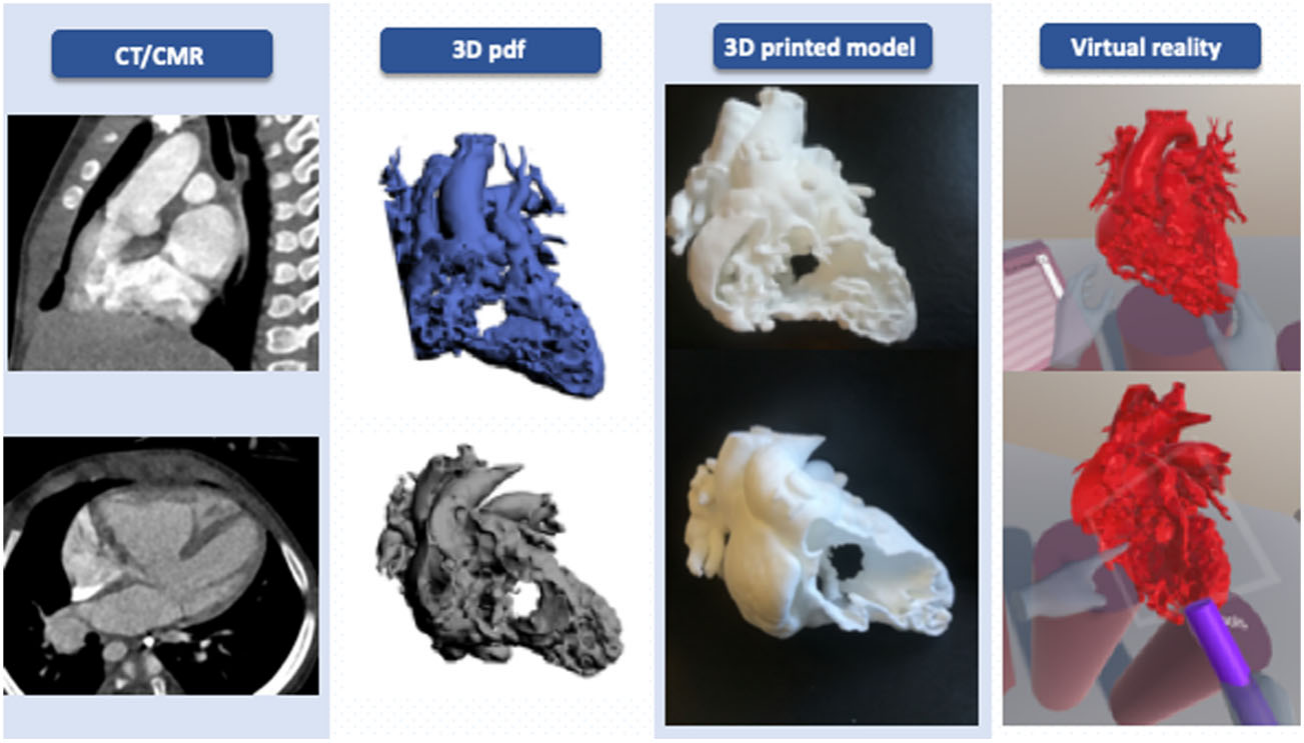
Two examples of three-dimensional visualization tools used by Surgeon A and B to assess the cases. CMR, cardiac magnetic resonance; CT, computed tomography; 3D, three-dimensional.
